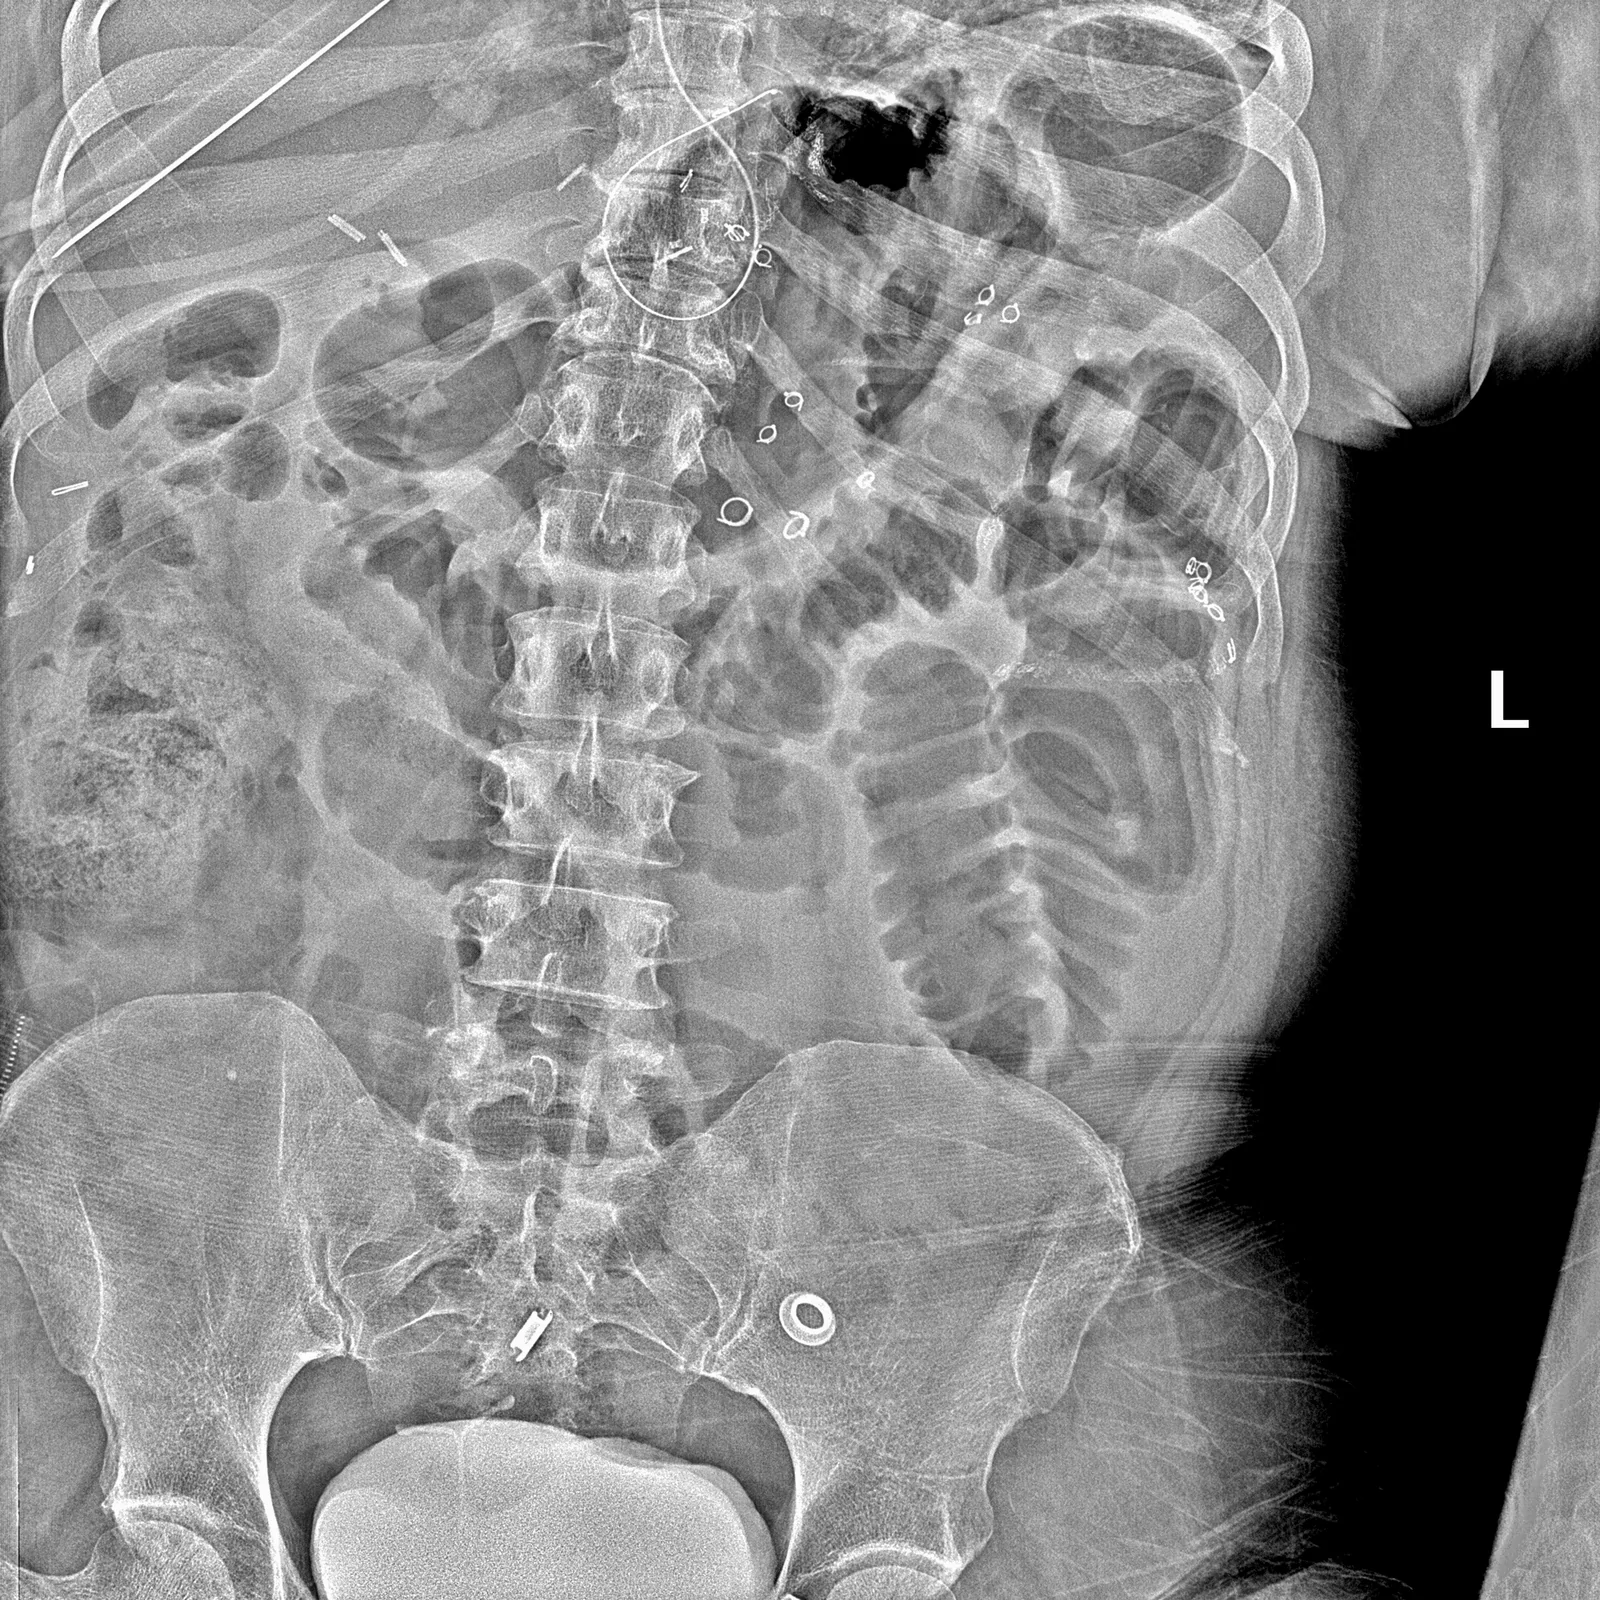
Postoperative abdominal radiograph confirming nasogastric tube placement, with the tip projecting over the gastric body.
